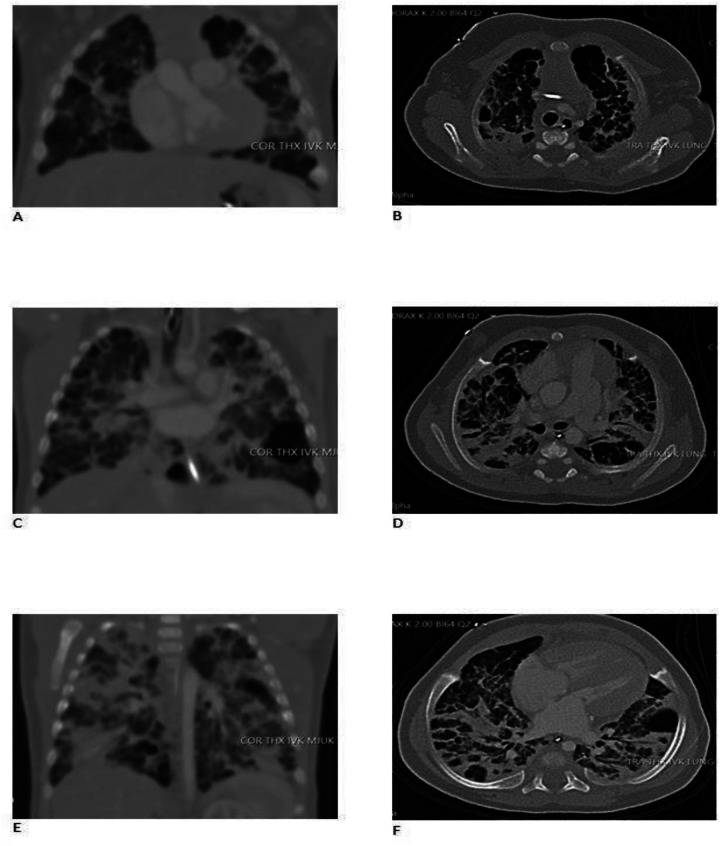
Ct scans with contrast of the chest. (A) CT (computed tomography) scan was performed at PMA (post-menstrual age) 41w before closure of the shunts. Frontal view on left, anterior to dorsal (A,C,E) and transverse view on right, apical to basal (B,D,F). Pulmonary venous obstruction was excluded and the PDA and ASD could be verified. Pronounced bilateral lung parenchymal abnormalities with crazy paving, ground glass, intralobular lines, thickening of interlobular septa, and dorsal consolidation were noticed in keeping with BPD. Consolidation of the middle lobe with no suspicion of intralobular sequestration was seen. Ventrobasal in the left lower lobe an 18 × 20 × 16 mm air filled cystic structure, probable a pneumatocele was diagnosed. Several small cystic changes were seen bilateral, especially in basal parts of the lung. A mild pulmonary edema could not be excluded.
